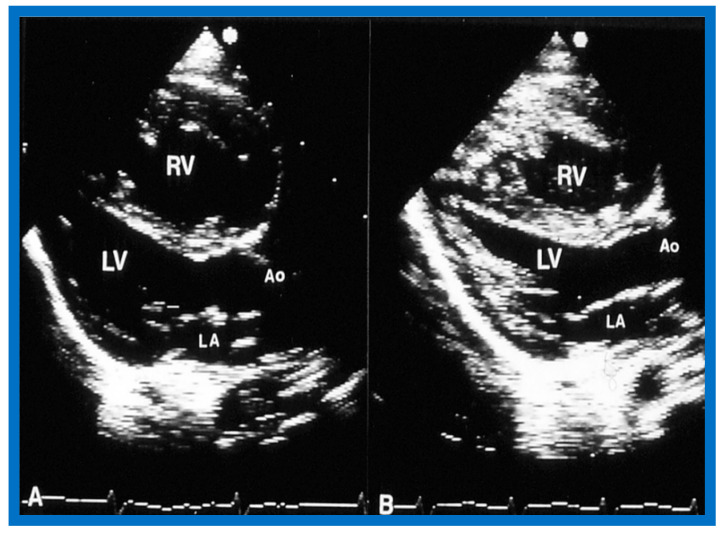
Selected video frames of two-dimensional images from parasternal long axis view prior to (A) and following (B) balloon pulmonary valvuloplasty, demonstrating a decrease in the size of the right ventricle (RV). Ao, aorta; LA, left atrium; LV, left ventricle. Reproduced from Reference [136].
